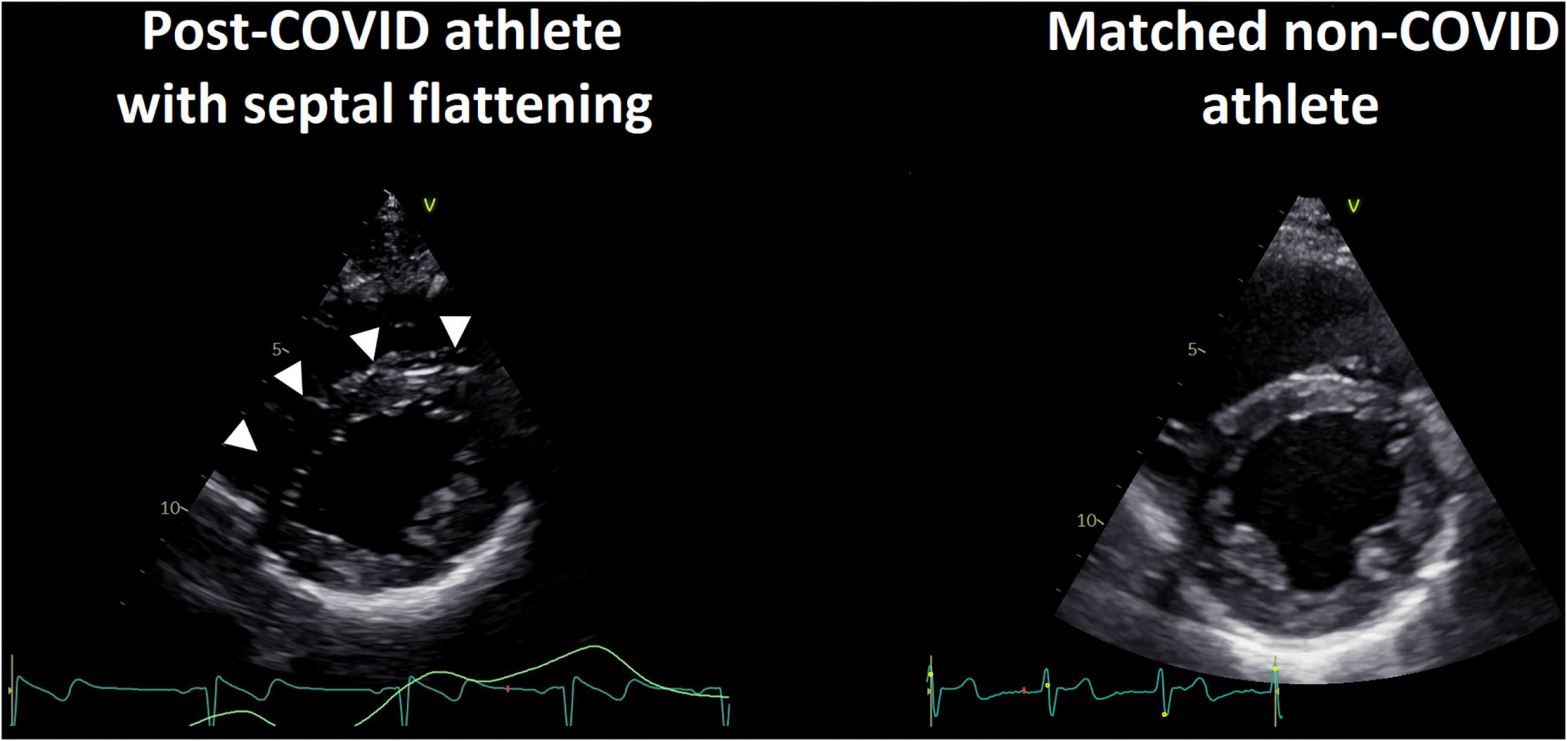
Representative case of the post-COVID septal flattening (SF) in athletes. Parasternal short-axis views at the level of the papillary muscles at mid-diastole in a young athlete underwent asymptomatic SARS-CoV-2 infection and his matched control. In the post-COVID athlete, a prominent SF can be seen with early diastolic dominance and inspiratory enhancement (left, SF shown by arrows), compared to the propensity score-matched control (right).
